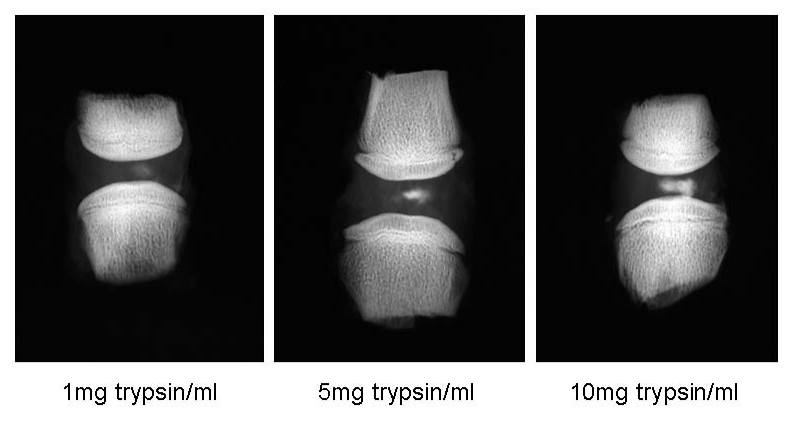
Radiographic images of motion segments injected with radio-opaque medium 72 hours after incubation with 1, 5 or 10 mg/ml trypsin.
